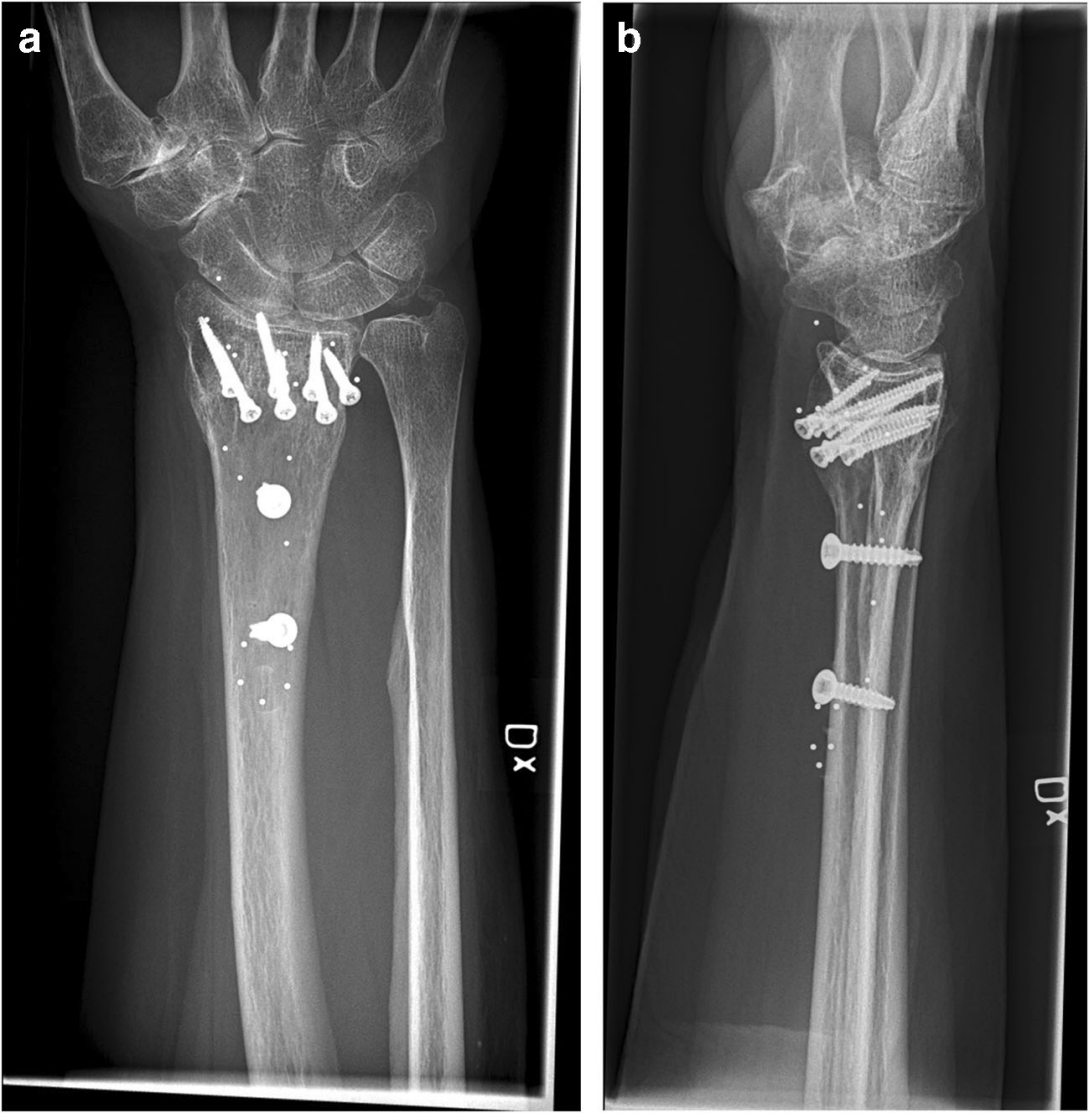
Radiographs at 12 months control after open wedge osteotomy. a AP view. b Sagittal view
